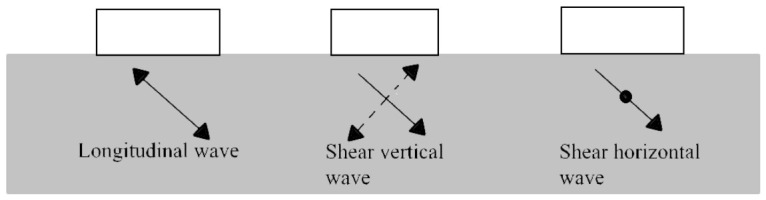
Polarization of ultrasonic bulk waves. Longitudinal (P) waves have particle motion parallel to the propagation direction, while shear vertical (SV) and shear horizontal (SH) waves have particle motion perpendicular to the propagation direction, in the vertical and horizontal planes respectively. Adapted from [2].
